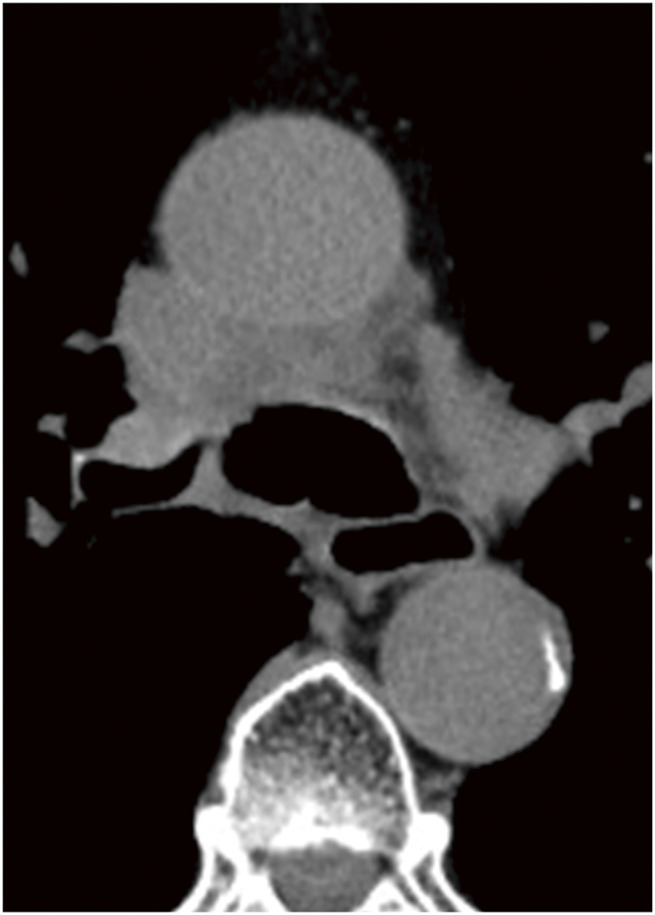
Non-contrast CT image obtained 4 months after the appearance of the fistula, at which time complete closure had been confirmed, showing marked regression of the pretracheal lesion with no evident residual cavity or mass. The status of the previously observed ring-like structure could not be assessed due to the lack of contrast enhancement. CT, computed tomography
